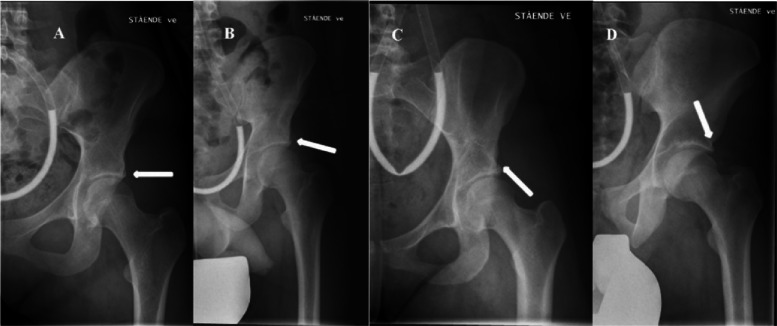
Radiographs of four participants with acetabular phenotypes classified according to latent class method. In the normal phenotype (A), the weight-bearing, bony area of the acetabulum, seen as a hyper-dense line along the lateral part of the acetabular roof, curves slightly downwards. This provides optimal coverage and support for the femoral head, without interfering with the femoral head-neck junction during movement. In hips with a tendency to AD (B) or with AD (C), the lateral part of the acetabulum has a more horizontal, or upward direction. In hips with over-coverage, also known as pincer-type FAI (D), the lateral part of the acetabulum has a more pronounced downward curve. This lateral extension can cause the acetabulum to interfer with the femoral head-neck junction during movement
